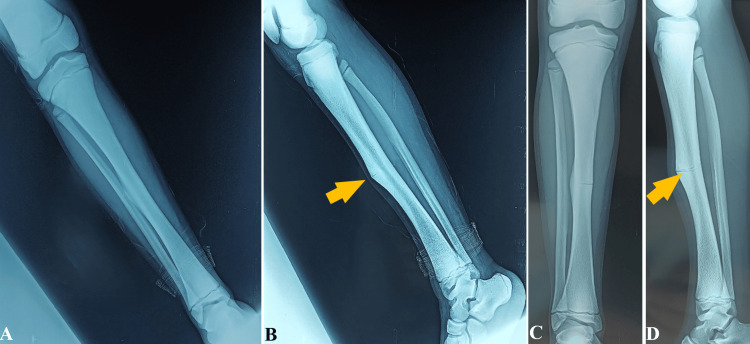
Lower-limb radiographs showing diaphyseal–metaphyseal sclerosis (A), a mid-diaphyseal tibial fissure with callus formation at the age of 15 years (arrow) (B), and a nondisplaced mid-diaphyseal fracture of the right tibia with prominent hypertrophic callus related to previous fractures at the same site (arrow) (C: frontal view; D: lateral view).
